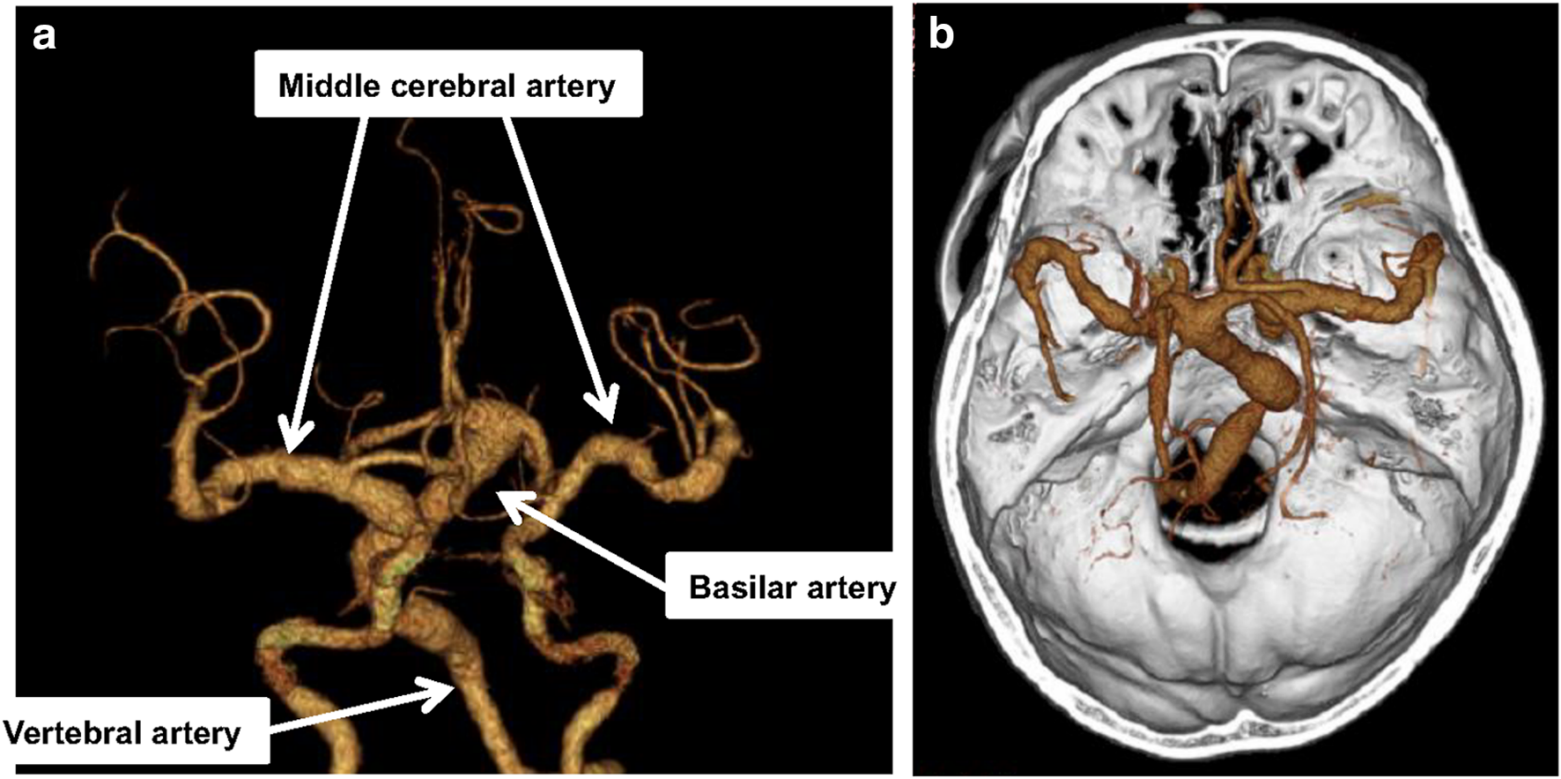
3D-CT scan image of the brain. CT scan image showed remarkable dilatation of the basilar, left vertebral, bilateral middle cerebral, and post cerebral artery. a Coronal view. b Axial view
